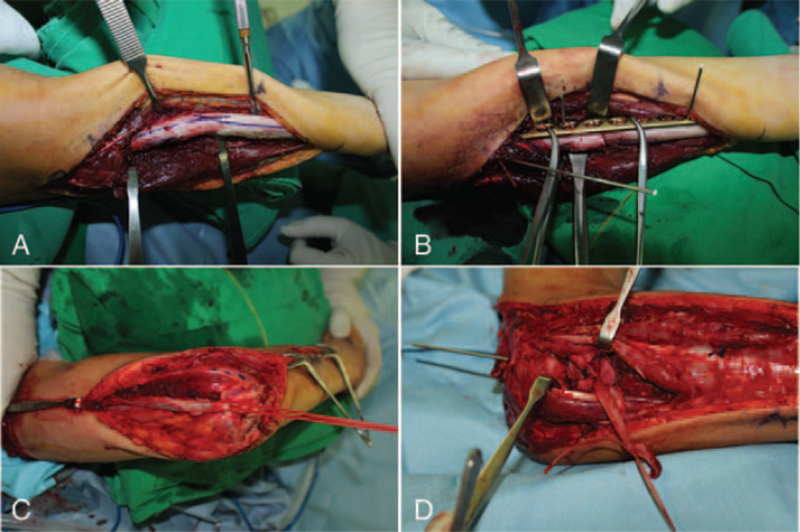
Preoperative radiographs performed at 16 mo after injury. Radiographs showing an anterior convex deformity of 20° and medial convex deformity of 21° at the radial fracture site indicated anterolateral dislocation of the radial head.
